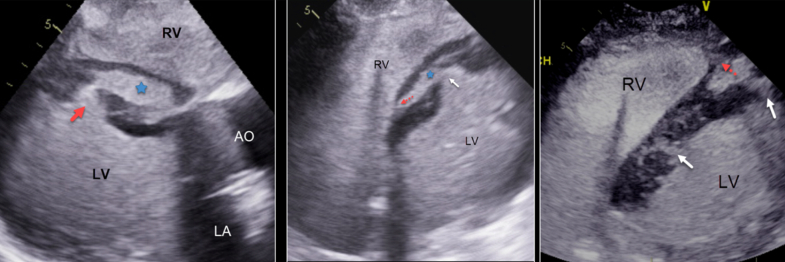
Contrast-Enhanced Images of the VSD and Dissections (Left) Parasternal long-axis view shows VSD inlet (red arrow) that leads to midseptal dissection cavity (blue star). (Middle) Off-axis 4-chamber view shows VSD opening (white arrow) into interventricular septum with dissection cavity (blue star) and exit toward RV base (red arrow). (Right) Off-axis 4-chamber view shows the VSD midseptal and apical entries (white arrows) and exit into the apical RV (red arrow). Contrast reveals a network of channels that dissect and infiltrate the infarcted myocardium. AO = aorta; LA = left atrium; LV = left ventricle; RV = right ventricle; VSD = ventricular septal defect.
